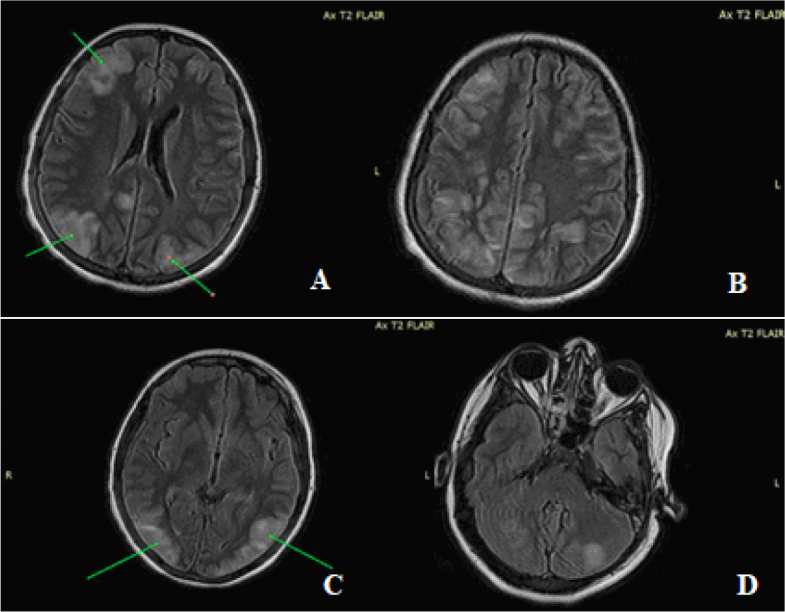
Multiple infra and supra centimeter lesions in hypersignal T2 and FLAIR diffusely arranged bihemispheric cortico-subcortical in (A, B) frontal and parietal lobes, (C) occipital lobe and (D) bihemispheric cerebellar
